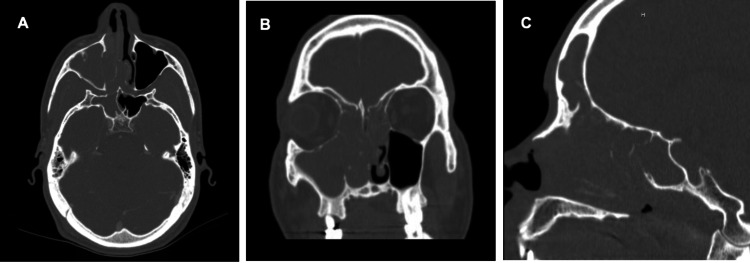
Computed tomography (CT) without contrast (A) Axial, (B) coronal, and (C) sagittal computed tomography (CT) without contrast showing a mass-like lesion occupying the paranasal sinuses, particularly involving the right maxillary, ethmoid, frontal and sphenoid sinus with extension into the right nasopharynx and left ethmoid sinus
